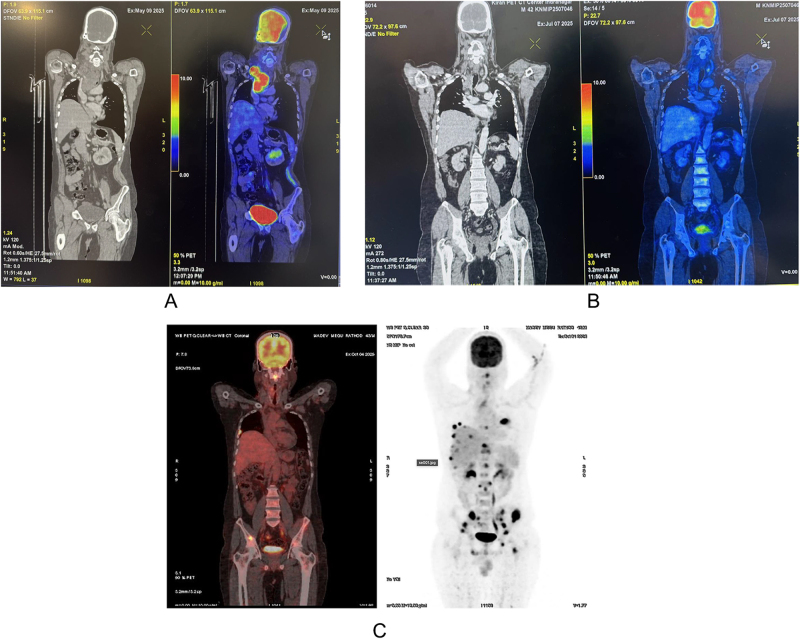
PET-CT evaluation of superior mediastinal SCC response to treatment. (A) Baseline PET-CT scan (before treatment) demonstrates an intensely FDG-avid lesion in the superior mediastinum, consistent with metabolically active nodal disease. (B) Follow-up PET-CT scan (after initial chemo-immunotherapy) shows near-complete resolution of FDG uptake in the mediastinum, suggestive of significant therapeutic response. (C) Latest PET-CT scan demonstrates multiple new FDG-avid hepatic, pleural, and skeletal lesions, indicating systemic metastatic progression despite prior locoregional response.
